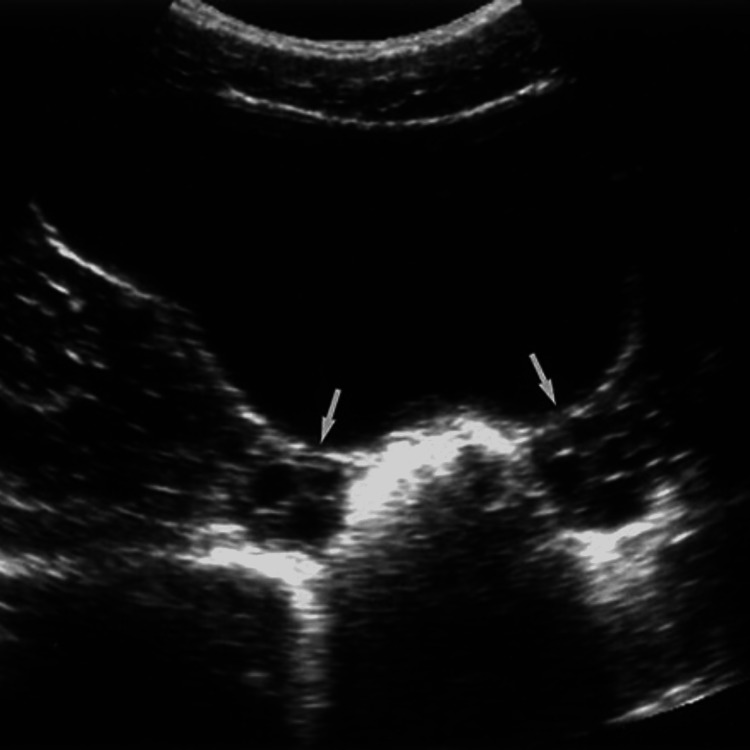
Effects of full urinary bladder during ultrasound scanning of clearly visualized the ovaries (arrows)
